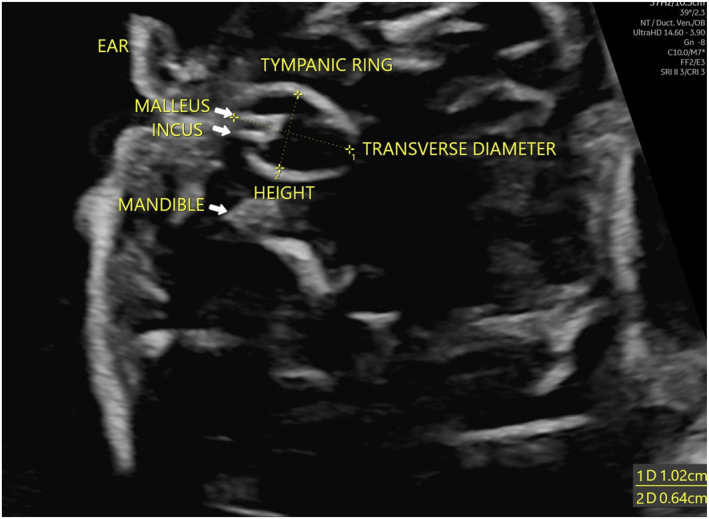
Normal tympanic ring—abdominal 2D ultrasound image of the axial section of the base of the brain to show the tympanic ring, the malleus and incus were in the lateral half of the ring, and the cross‐section of the mandible ramus beneath the ring. The transverse diameter and height were measured by placing the pointers at the inside border of the ring.
